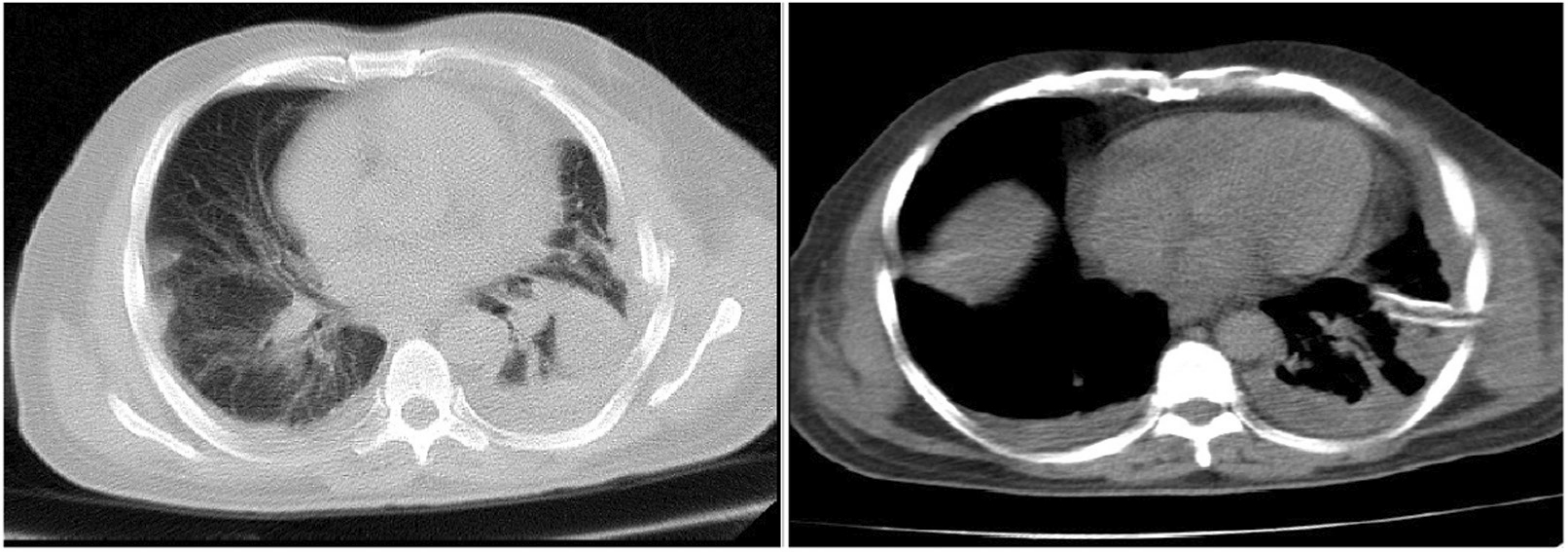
Axial views of the chest HRCT scan illuminating peripheral bilateral pleural effusion and ground glass opacities for COVID‐19
